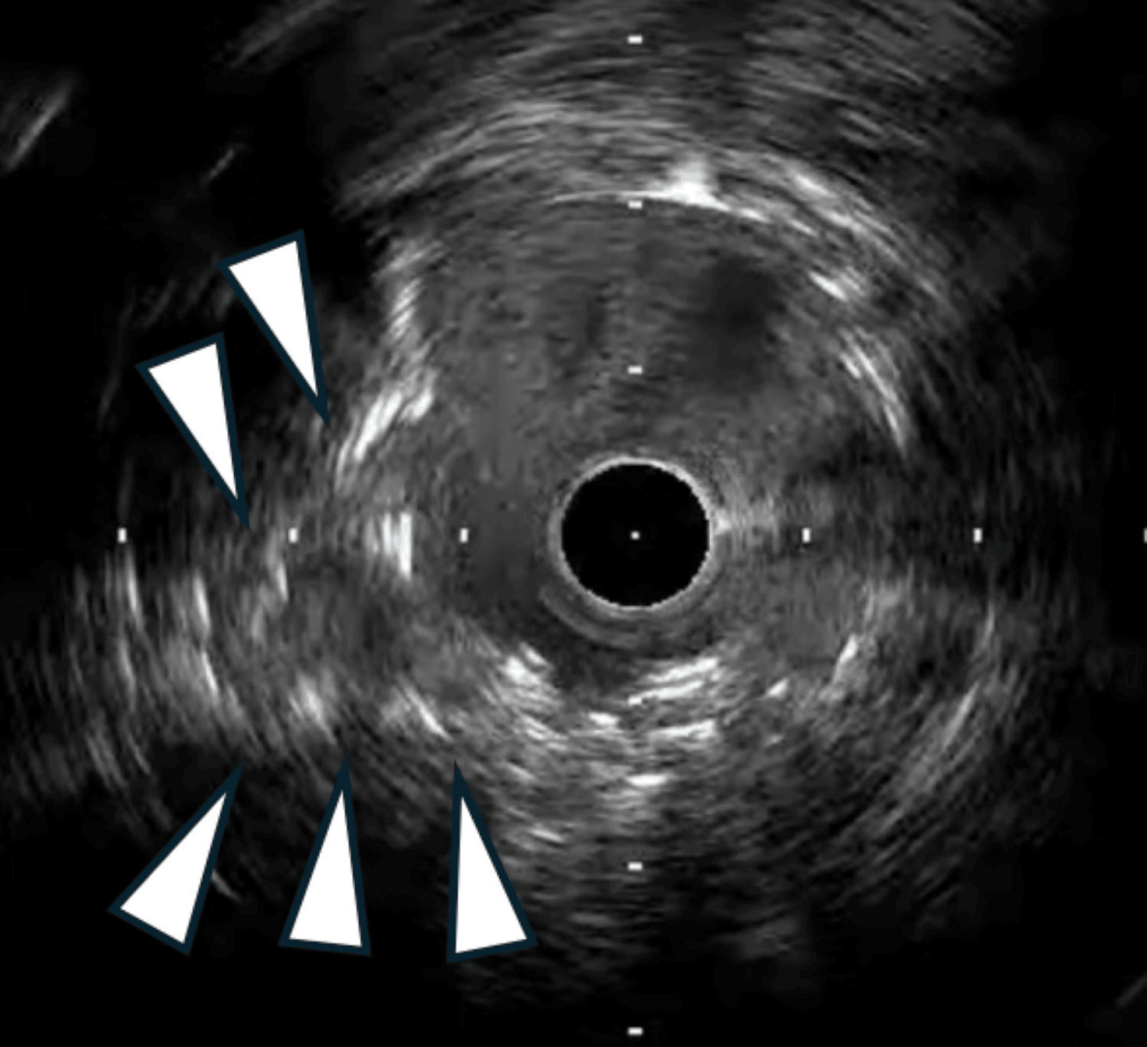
Intravascular ultrasound confirmed compressing stent nodule (white arrowhead)
